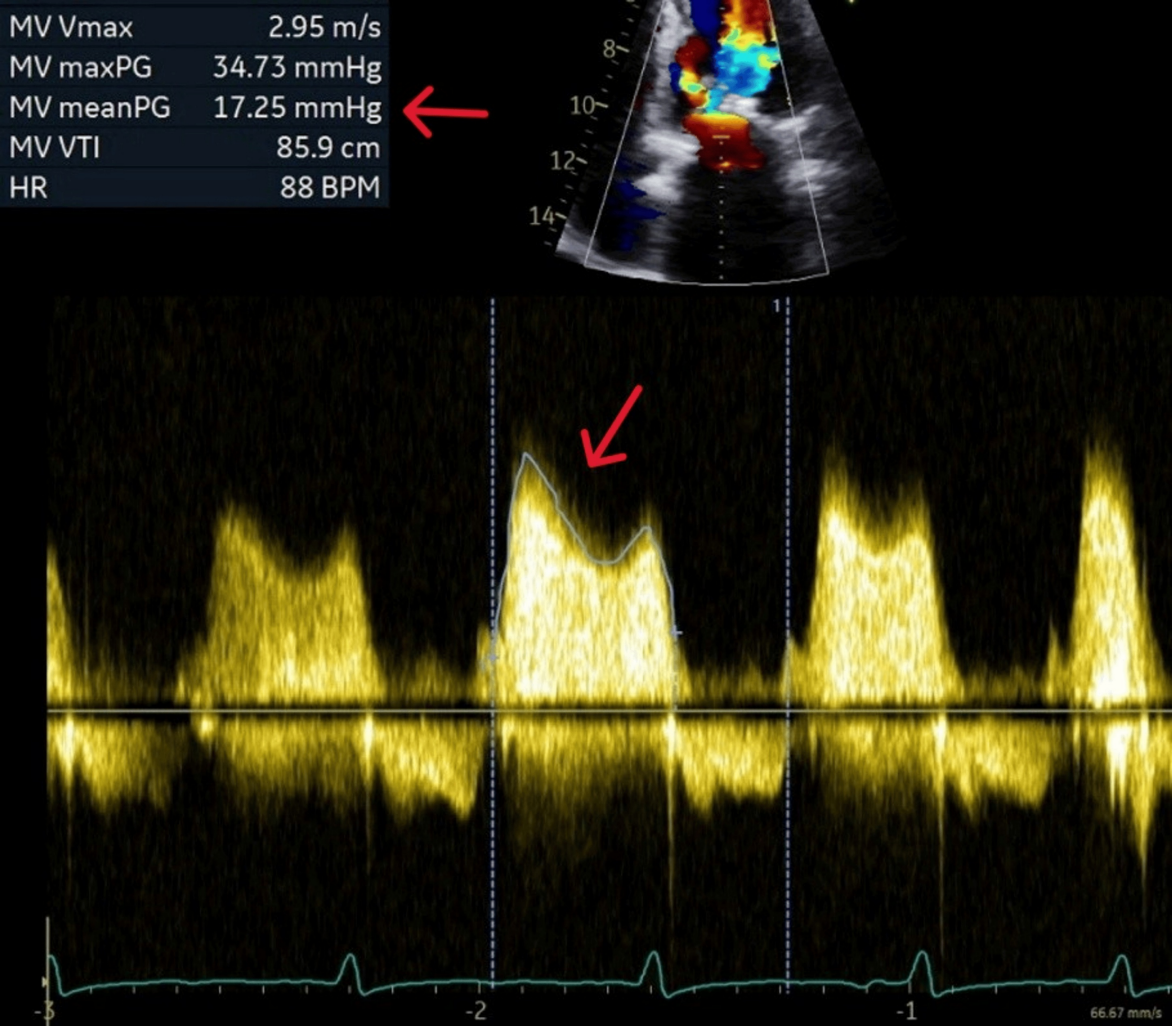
Transthoracic echocardiography, continuous wave doppler showing high mean pressure gradient (arrows).
